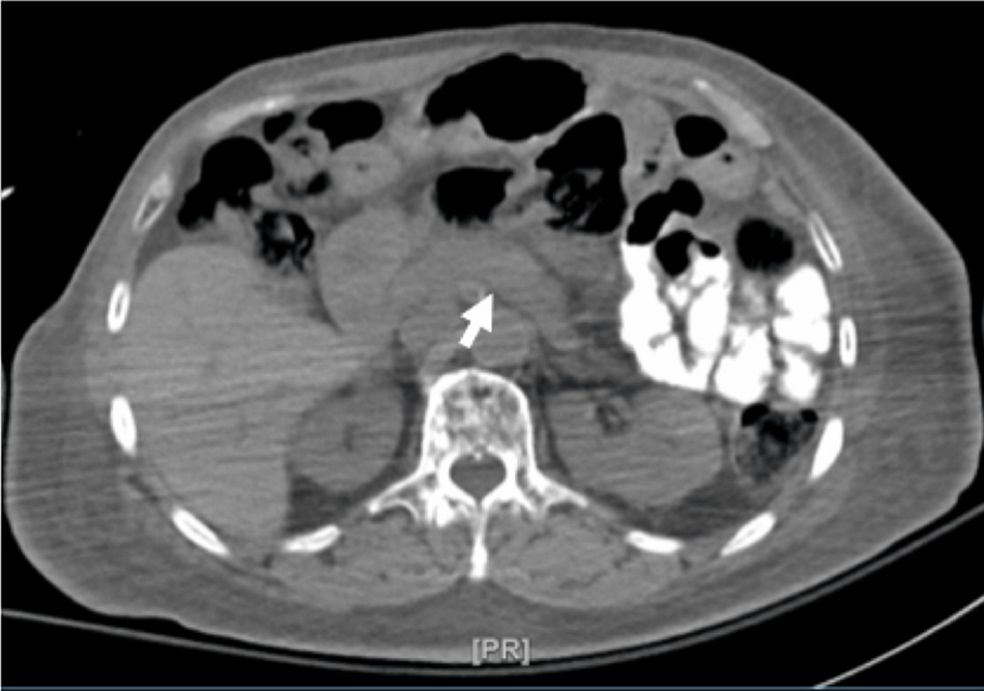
Axial CT scan of the abdomen showing normal appearance of the pancreas (white arrow) CT: computed tomography
